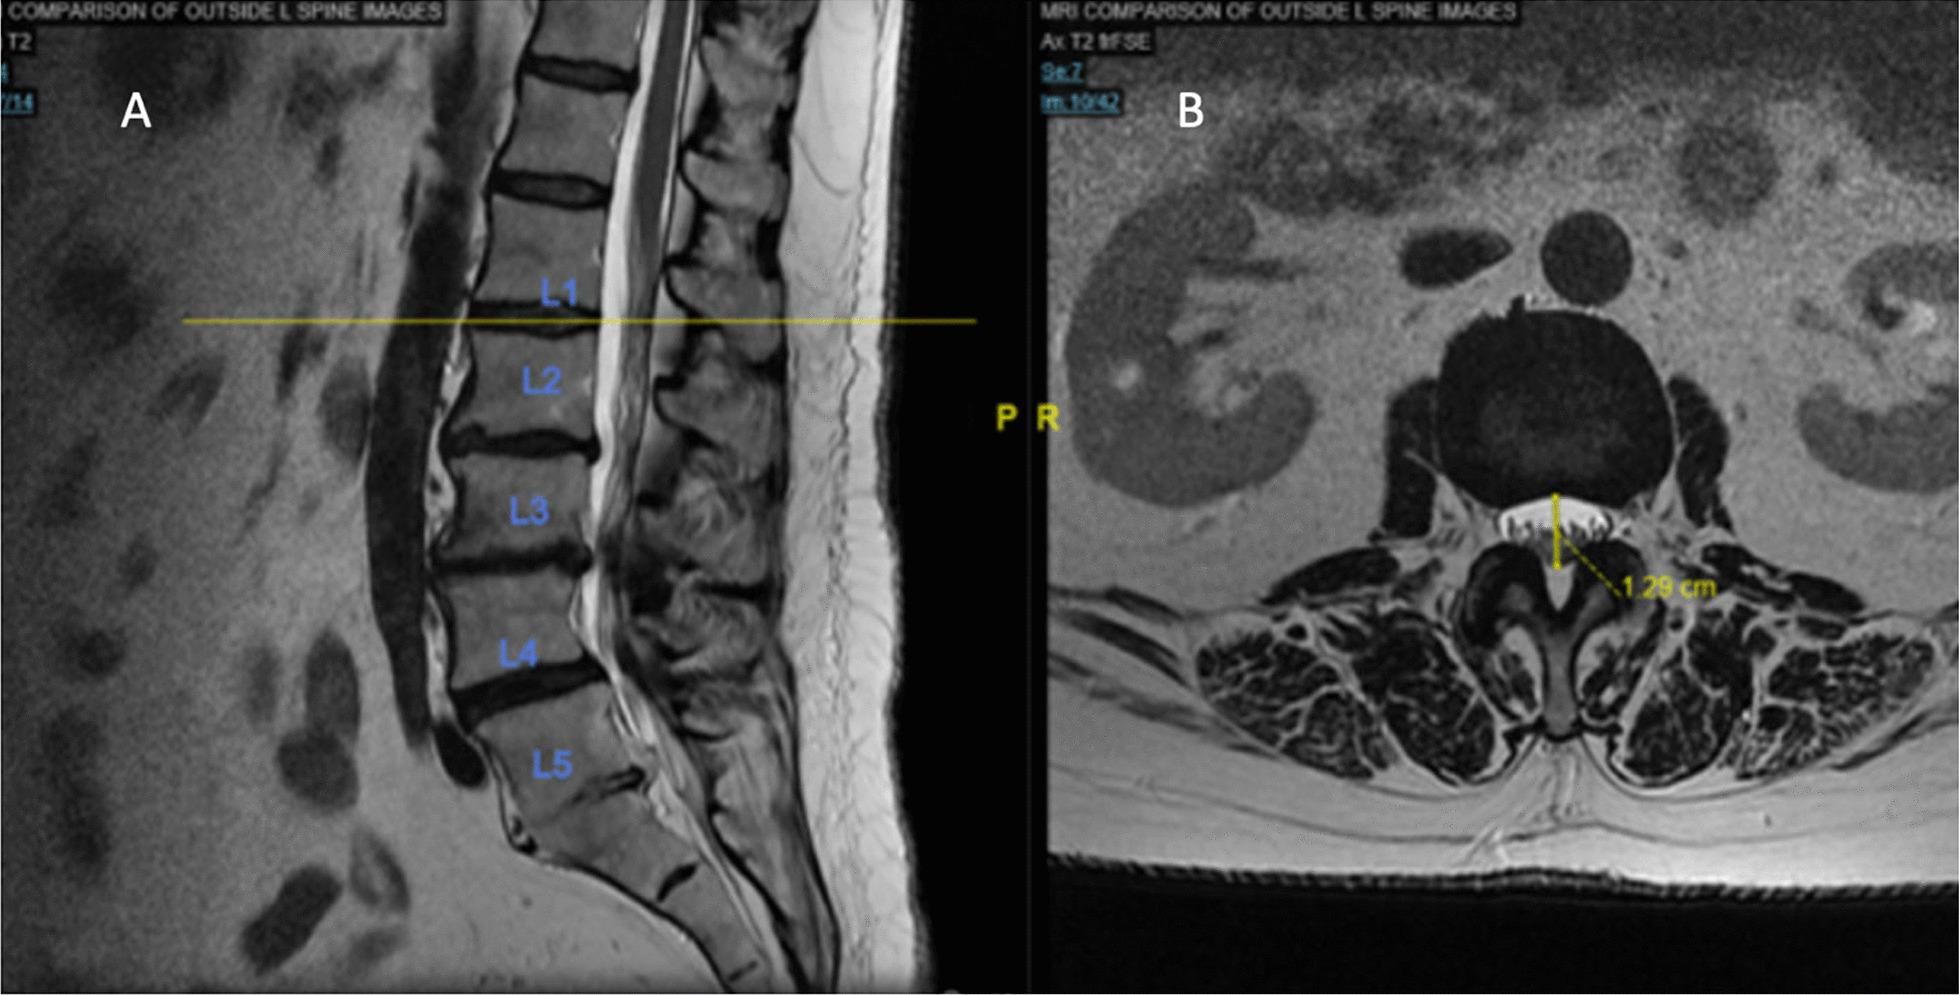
Anterior–posterior (AP) diameter. A Sagittal T2-weighted MRI image at the intervertebral disc space between lumber level 1 and 2. B The corresponding T2-weighted axial MRI image from which the AP diameter measurement is shown. A line drawn from anterior-most aspect of the thecal sac to the most posterior aspect yields the measurement needed to assess stenosis in the AP plane
